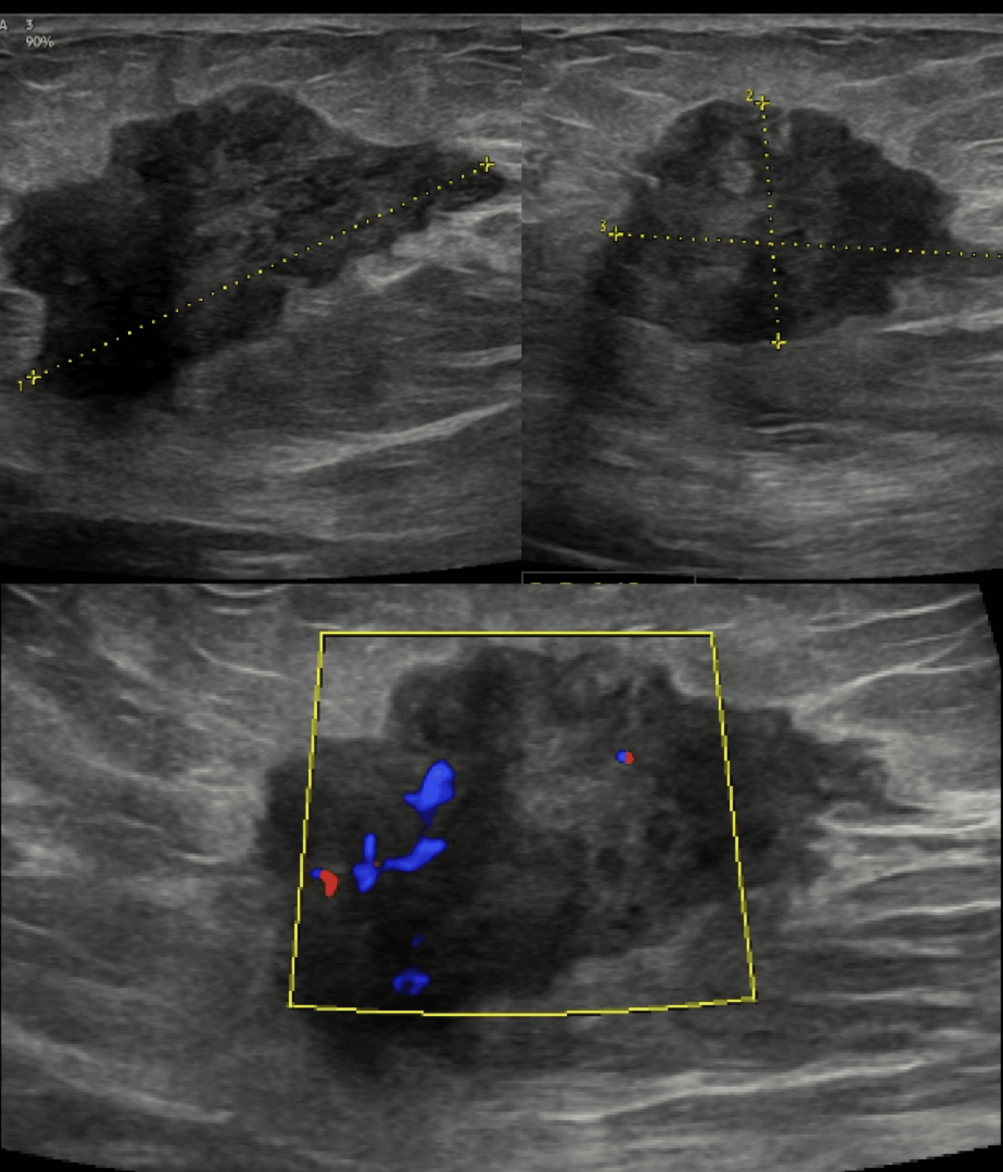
Ultrasound shows a spiculated hypoechoic mass with internal vascularity and surrounding architectural distortion.
